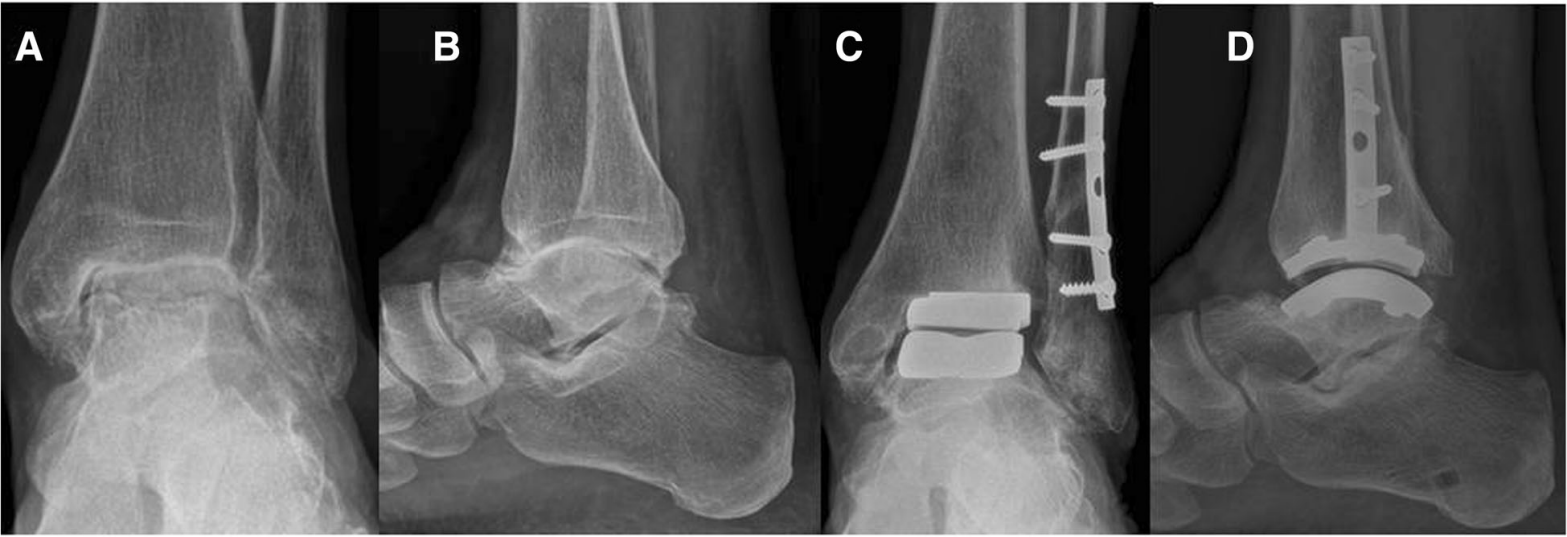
Pre-operative (a, b) and two-year follow-up weight bearing radiograph after Zimmer TM TAR showing a medial malleolar cyst (c, d)
